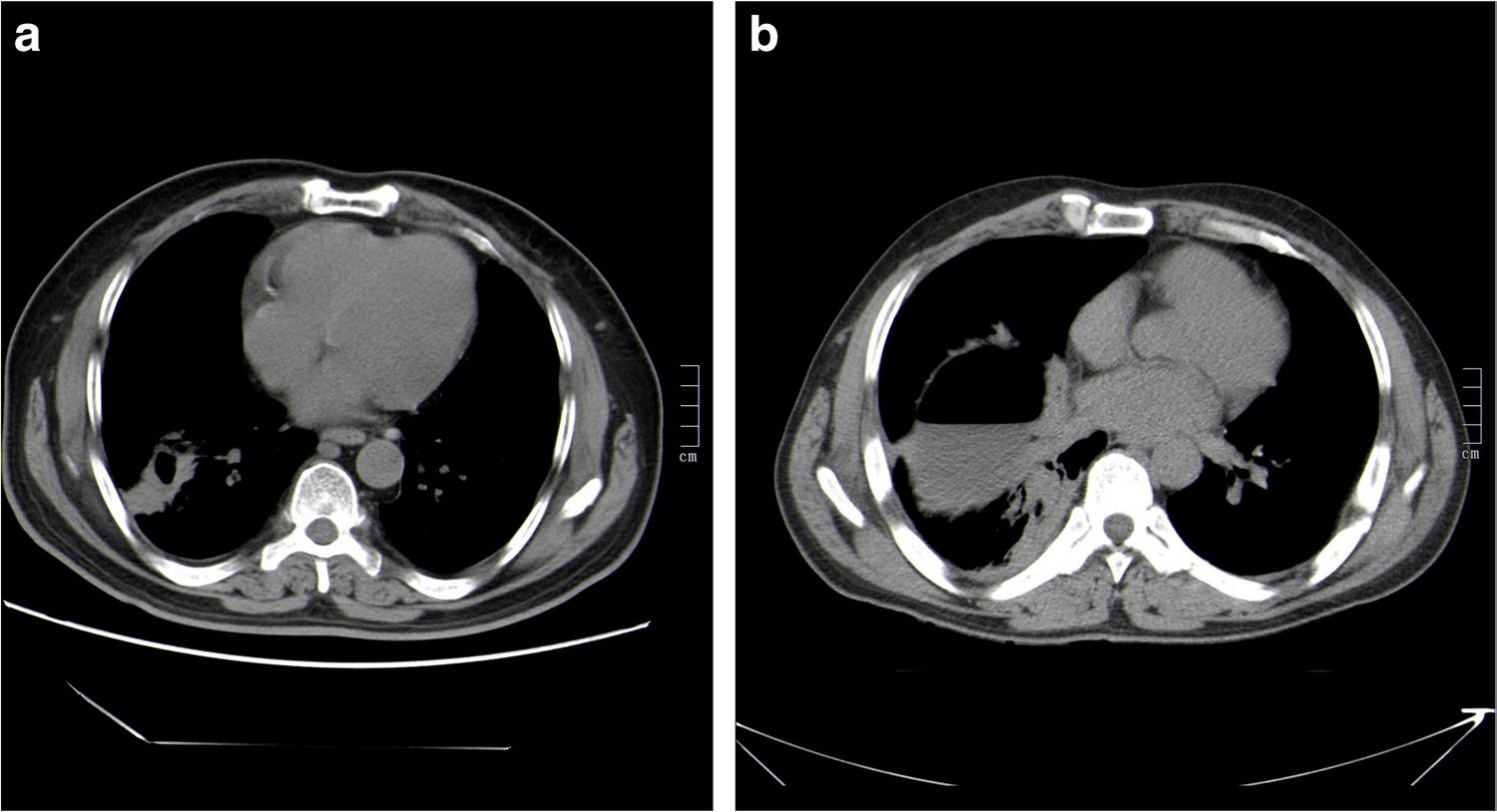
CT images of a pulmonary CE and b pulmonary abscess
